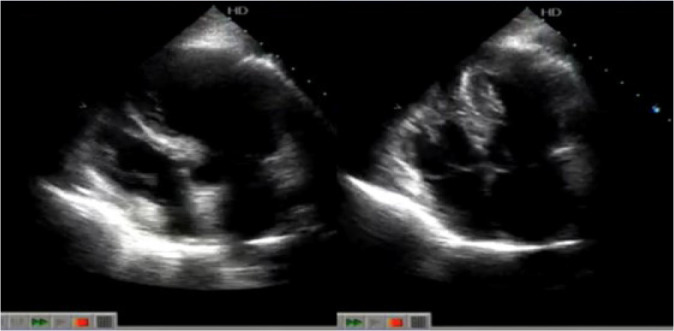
Echocardiogram (diastole and systole) from November 25, 2018 showing abnormality in the left ventricle, with the typical Takotsubo format.
